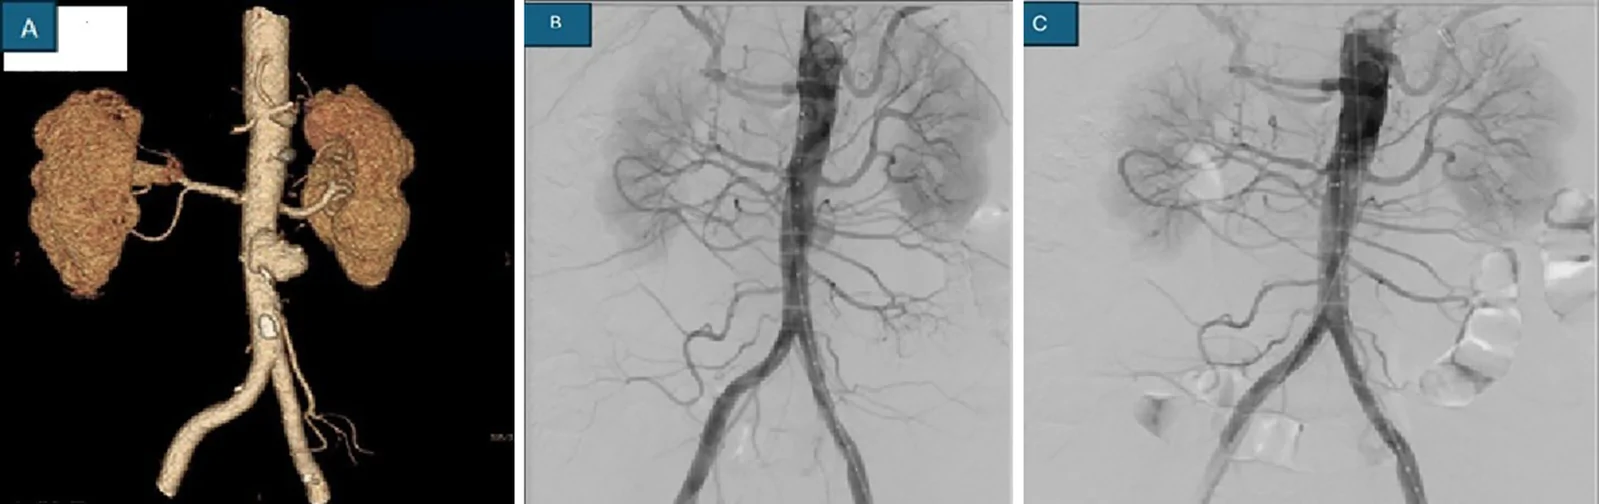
A) Pre procedure: CT angiogram of intra-abdominal aorta aneurysm. B) Pre procedure: Aortogram showing infrarenal abdominal aortic saccular aneurysm. C) Post procedure: Aortogram showing no filling of the aneurysm.
